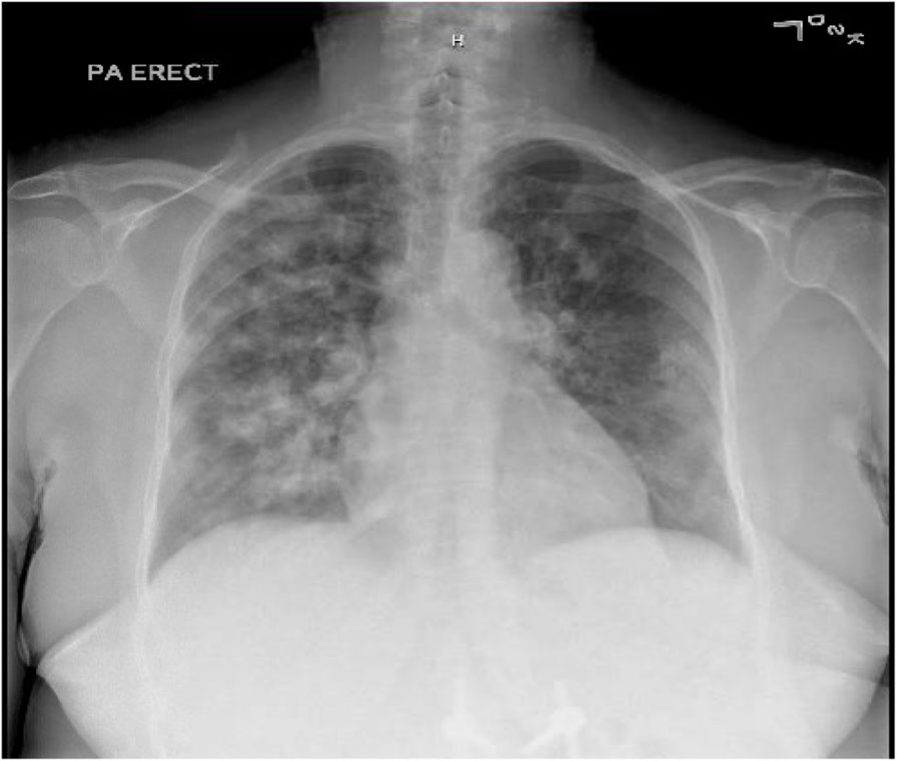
Chest X-ray shows diffuse nodular densities bilaterally but more prominent on the right lung
